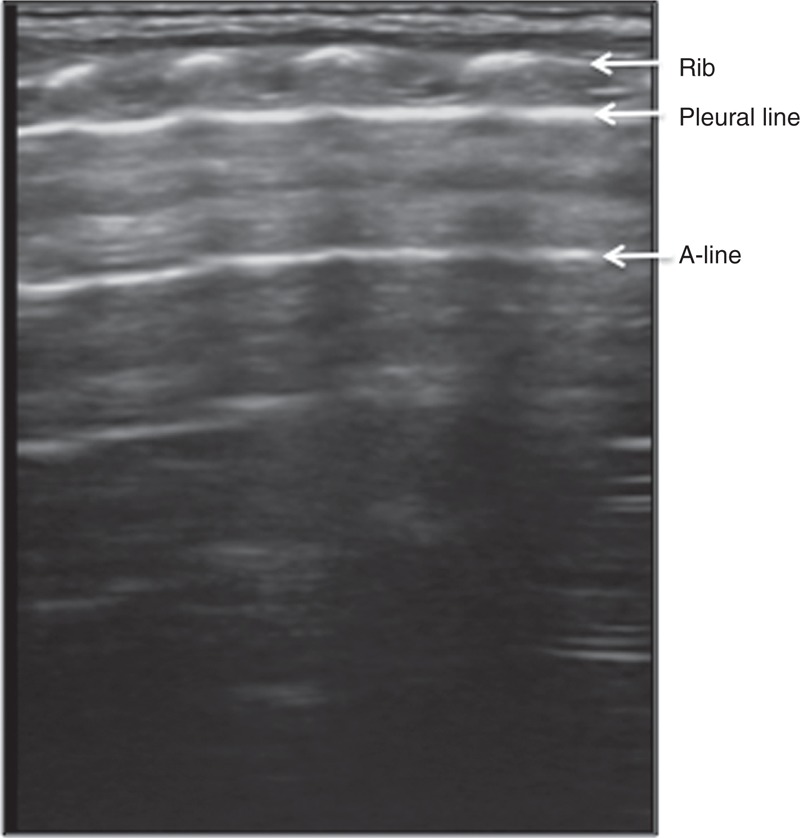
Normal neonatal lung ultrasonic manifestations. The normal neonatal lung ultrasonic manifestations were as follows. The lung tissue was overall hypoechoic, with pleural lines and A-lines that were smoothly, clearly and regularly hyperechoic. There were no manifestations such as interstitial lung syndrome, pulmonary consolidation, double-lung point, or pleural effusion.
